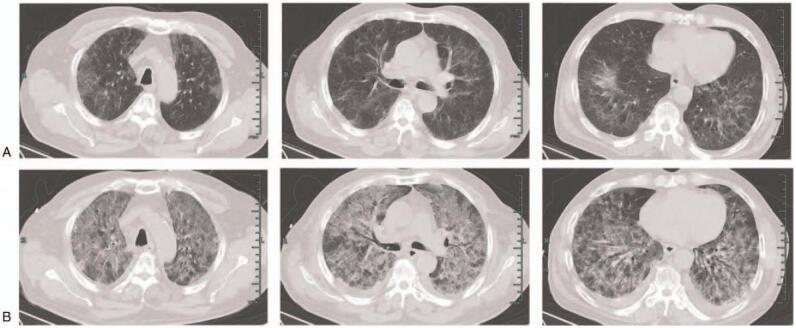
CT scan of the lung. A: On the 1st day, a CT scan showed extensive interstitial pneumonitis of 2 lung fields with the crazy-paving pattern in the middle and peripheral region of 2 upper lungs. B: On the 4th day, a CT scan showed the obvious deterioration of exudation and pleural effusion in both lungs.
